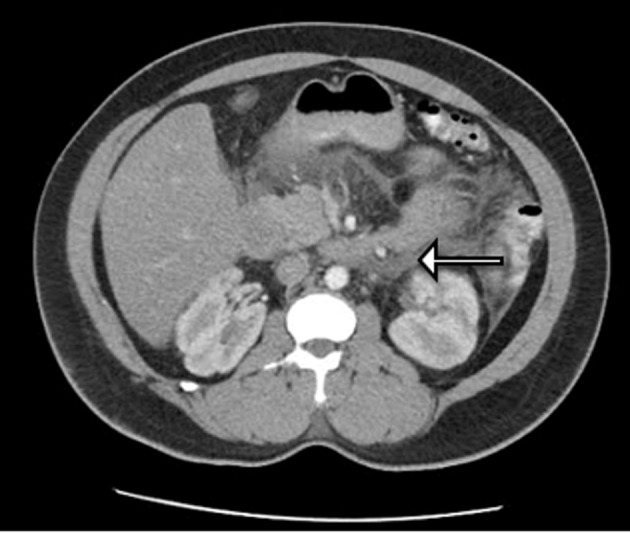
CT of abdomen demonstrating degree of pancreatitis.
